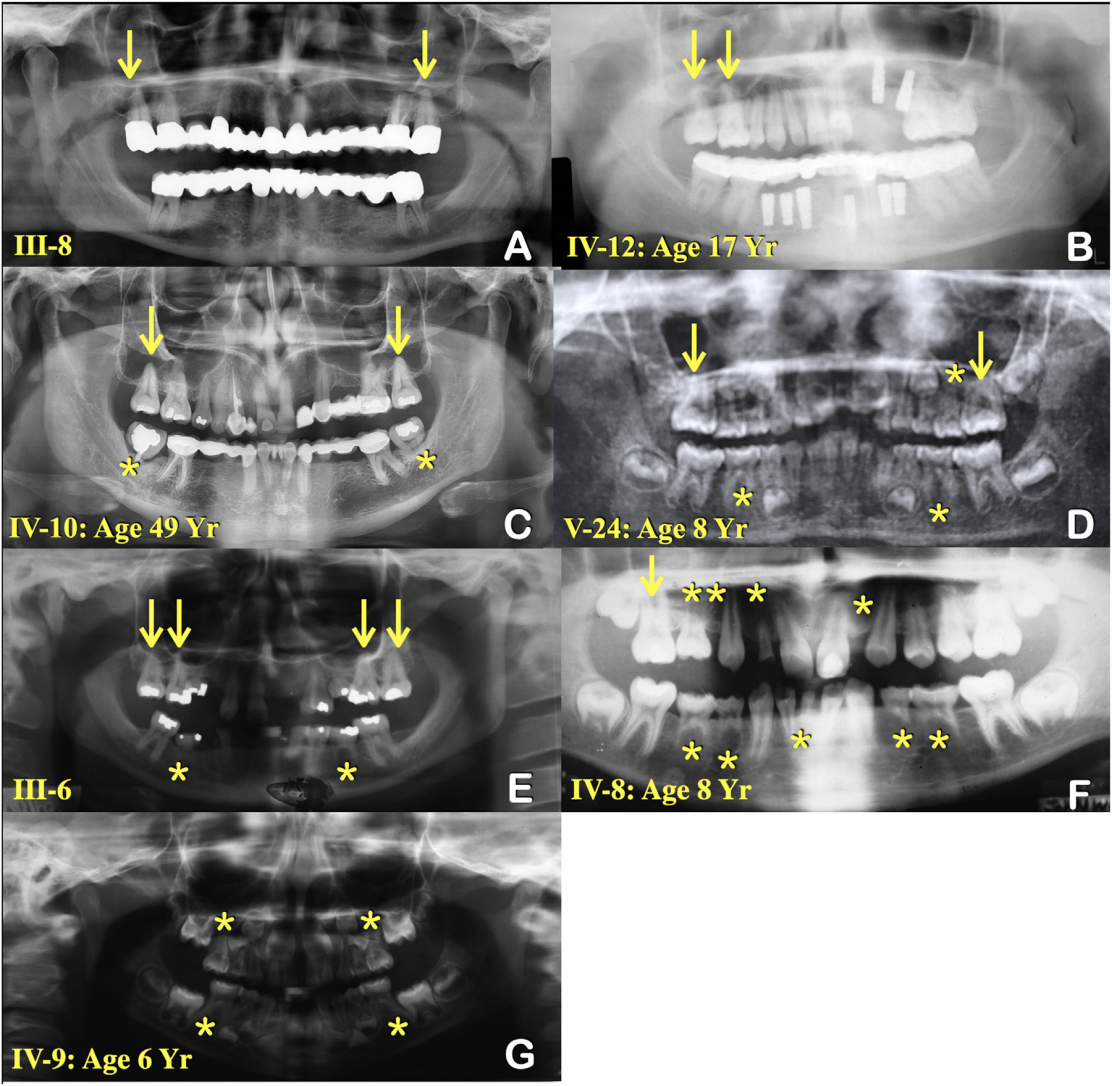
Panoramic radiographs of patients with the *KDF1* variant. (A) Patient III-8. Prosthetic bridges in the areas of tooth agenesis. Unseparated roots of the permanent molars (arrows). (B) Patient IV-12. Dental implants in the areas of tooth agenesis. Unseparated roots of the permanent molars (arrows). (C) Patient IV-10. Prosthetic bridges in the areas of tooth agenesis. Unseparated roots of the permanent molars (arrows). Rootless teeth (asterisks). (D) Patient V-24. Tooth agenesis (asterisks). Taurodontism (arrows). (E) Patient III-6. Tooth agenesis (arrows). Unseparated roots of the permanent molars (arrows). (F) Patient IV-8. Tooth agenesis (asterisks). Taurodontism (arrows). (G) Patient IV-9. Tooth agenesis (asterisks).
